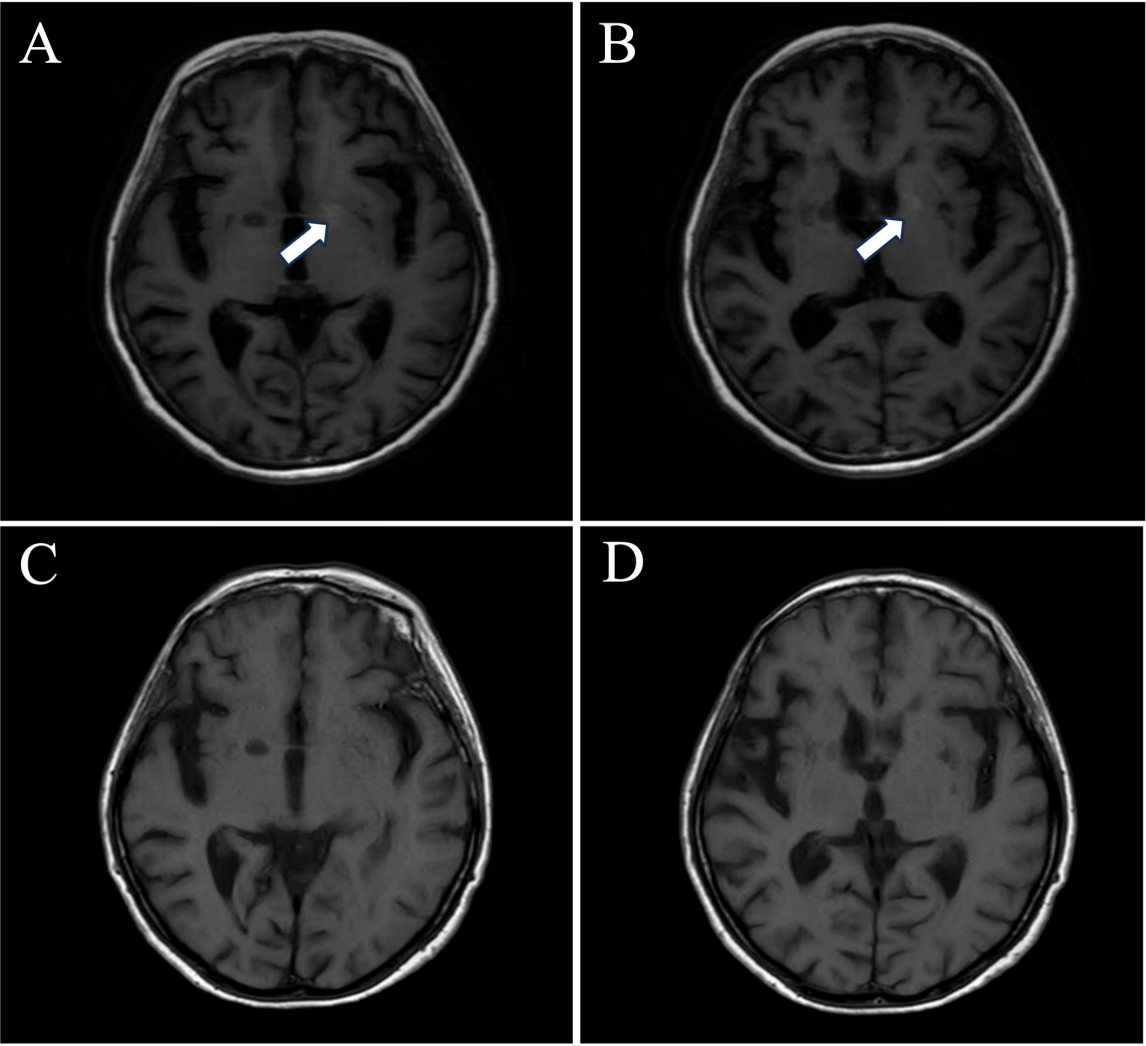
Brain MRI of case 3 showed T1-weighted hyperintensities in the left basal ganglia (A, B, marked by white arrows). The hyperintense signal on T1-weighted imaging in the left basal ganglia has resolved (C) after 44 months of follow-up.
